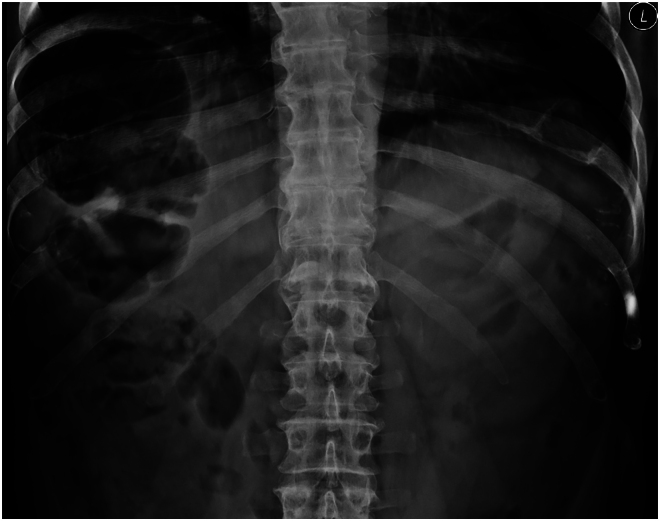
X-ray showing caecal volvulus
